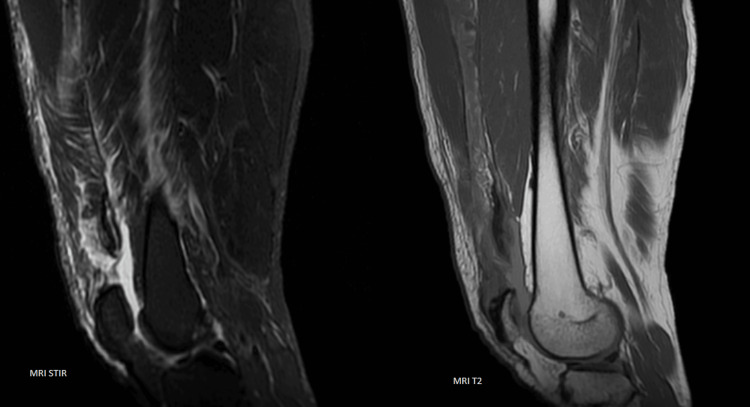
MRI scan (STIR and T2 views) MRI, magnetic resonance imaging
